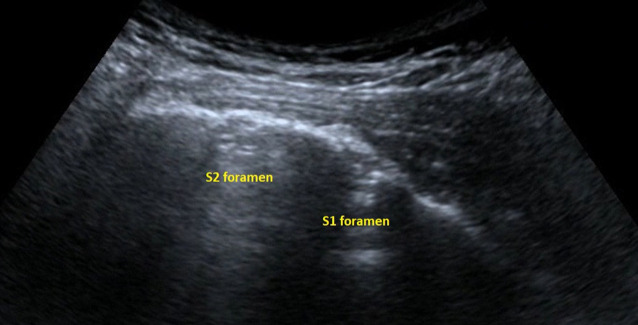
Ultrasound images of the S1 and S2 foramen.
